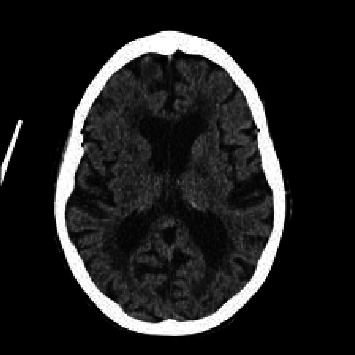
A noncontrast axial cranial CT image of the patient showing dilated sulcal spaces consistent with age-related brain atrophy and no other abnormalities.
